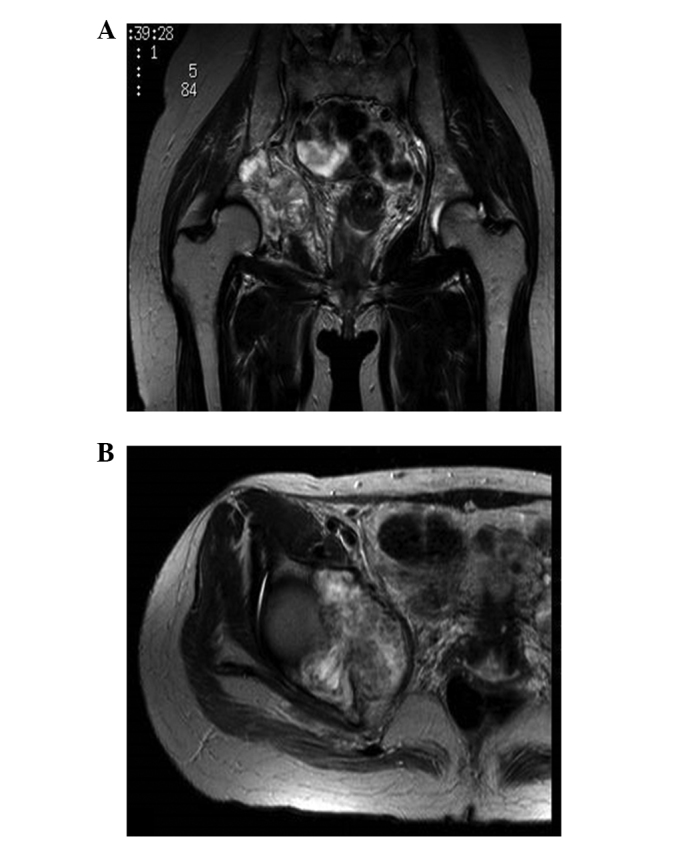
Case one: (A) Coronal and (B) axial T2-weighted magnetic resonance imaging (MRI) showing a mass with inhomogeneous intensity located in the right acetabulum.
